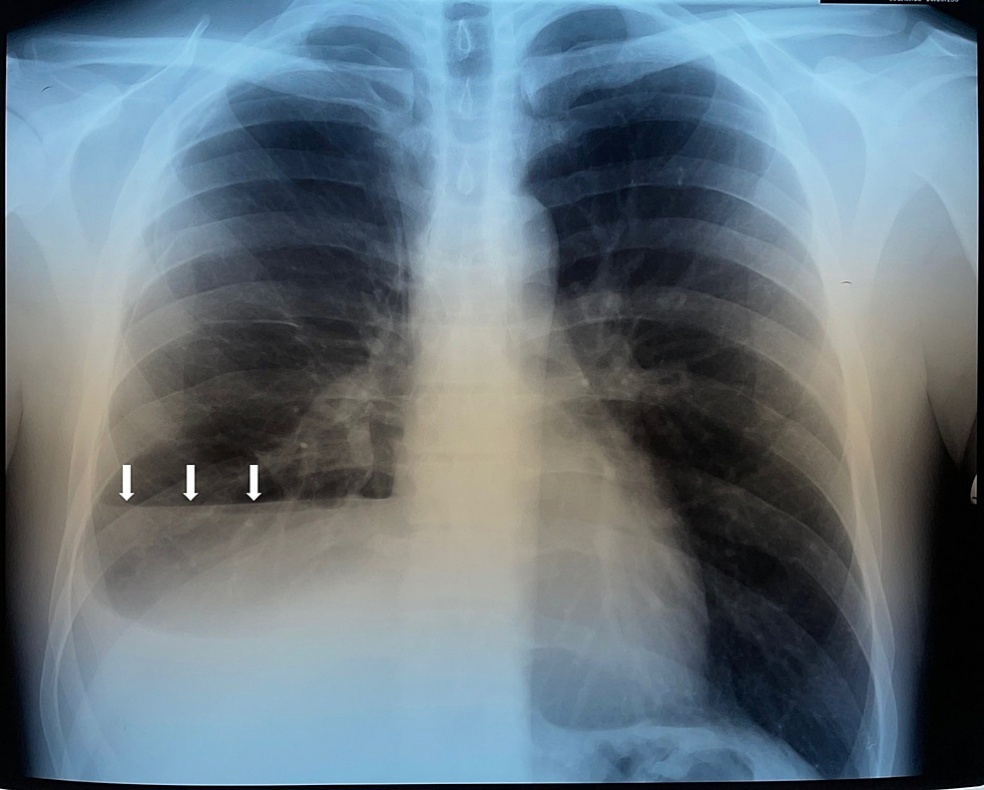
Initial chest x-ray showing air fluid level in the right thoracic cavity (white arrows)
